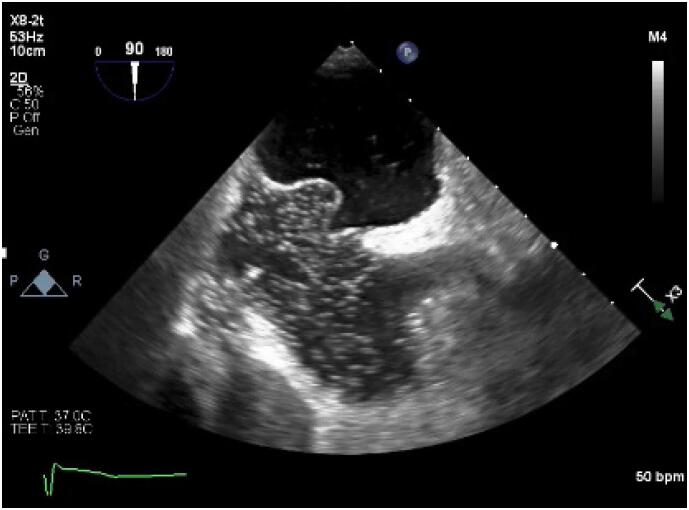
Transesophageal echocardiogram showing an aneurysmal interatrial septum with bubble passage from the right atrium to the left atrium
